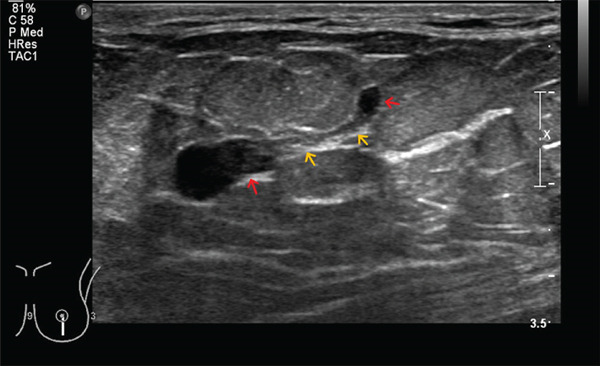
Ultrasound of the left breast at the 6 o′clock position in radial projection demonstrates two adjacent complex cystic and solid masses (red arrows), with intraductal solid tissue extending between them (yellow arrows), corresponding to the anterior mammographic findings in Figure 6. BI‐RADS 4 (suspicious; biopsy recommended).
